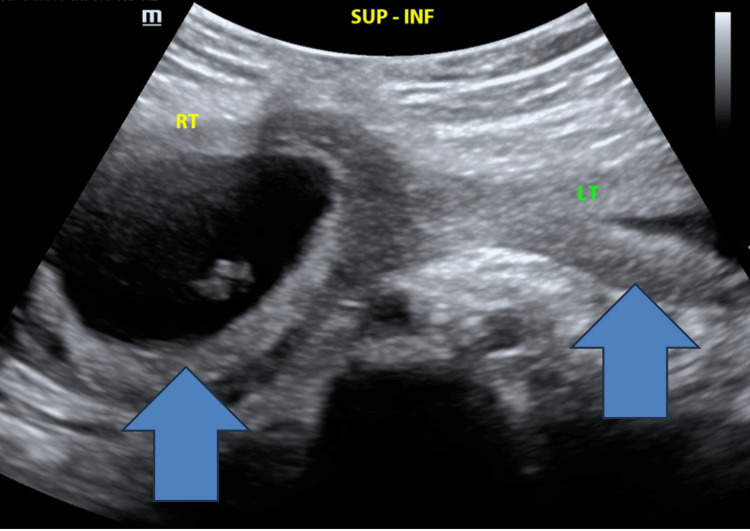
Transabdominal pelvic ultrasound at eight weeks' and five days' gestation demonstrating uterine didelphys. A gestational sac with yolk sac is visualized within the right hemi-uterus. A second, separate uterine cavity consistent with the left hemi-uterus is also visualized without evidence of pregnancy. Arrows indicate the gestational sac within the right hemi-uterus and the separate left hemi-uterus. These findings are consistent with uterine didelphys (original clinic image).
